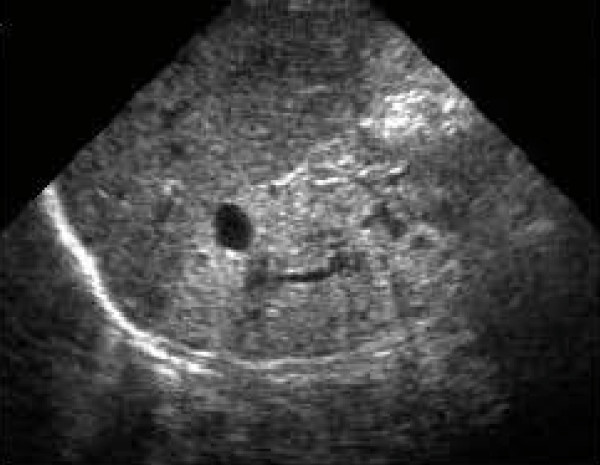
Renal ultrasonography. Bilateral renal enlargement and increased renal parenchymal echogenicity.
